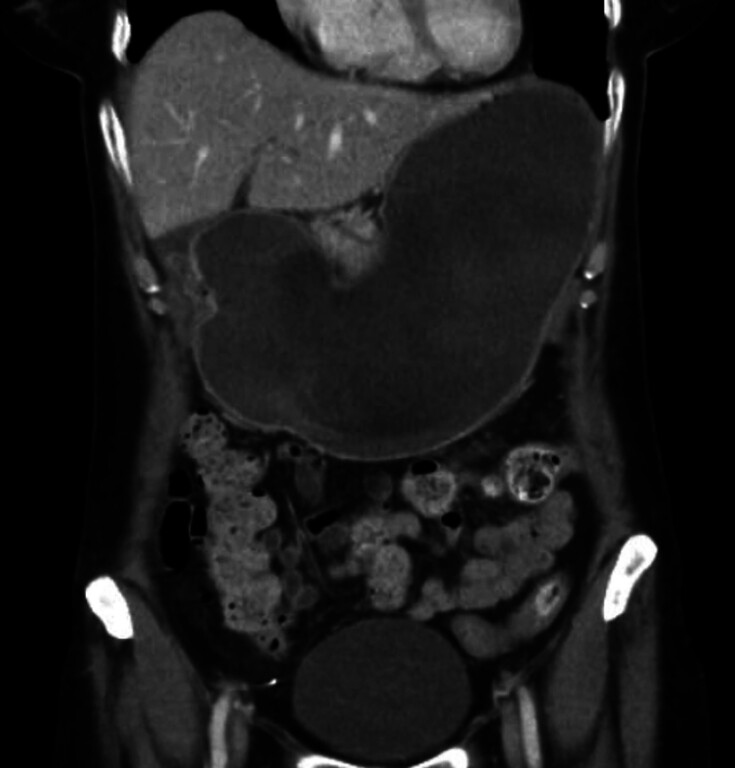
Computed tomography image (coronal) showing the distended, fluid-filled stomach.
